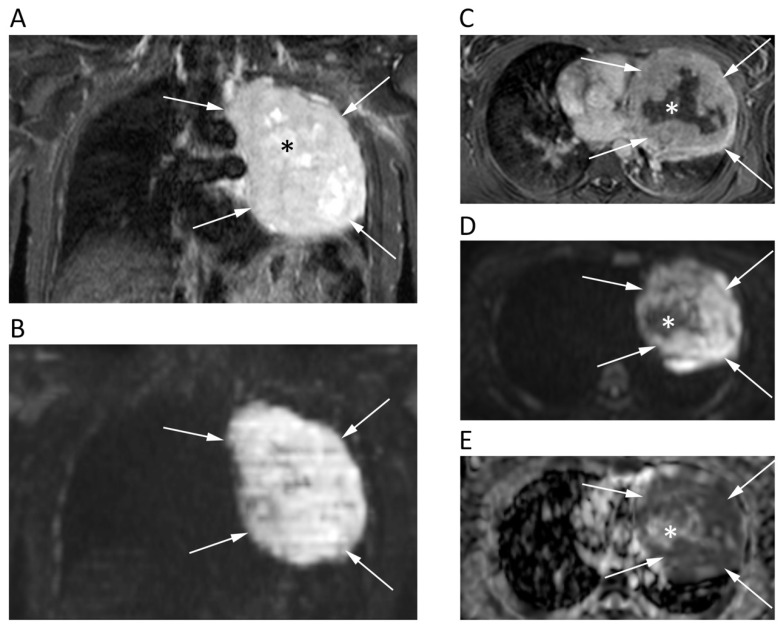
Chest MRI of a 62-year-old male patient with lung carcinoma. The coronal short tau inversion recovery (STIR) (A), coronal reconstruction of b 1000 DWI image (B), axial post-contrast T1w image (C), axial *b* = 1000 DWI image (D), and corresponding ADC map (E) show a large tumor in the left lung (arrows) with an restricted pattern of diffusion. Note the central necrotic areas (asterisks) presenting fluid signal intensity on STIR (A), absent contrast enhancement (C) and unrestricted pattern of diffusion (D,E) with mean ADC of 2.168 × 10^−3^ mm^2^/s. Conversely, the solid component of the tumor shows strong contrast enhancement and very low ADC values (0.818 × 10^−3^ mm^2^/s).
